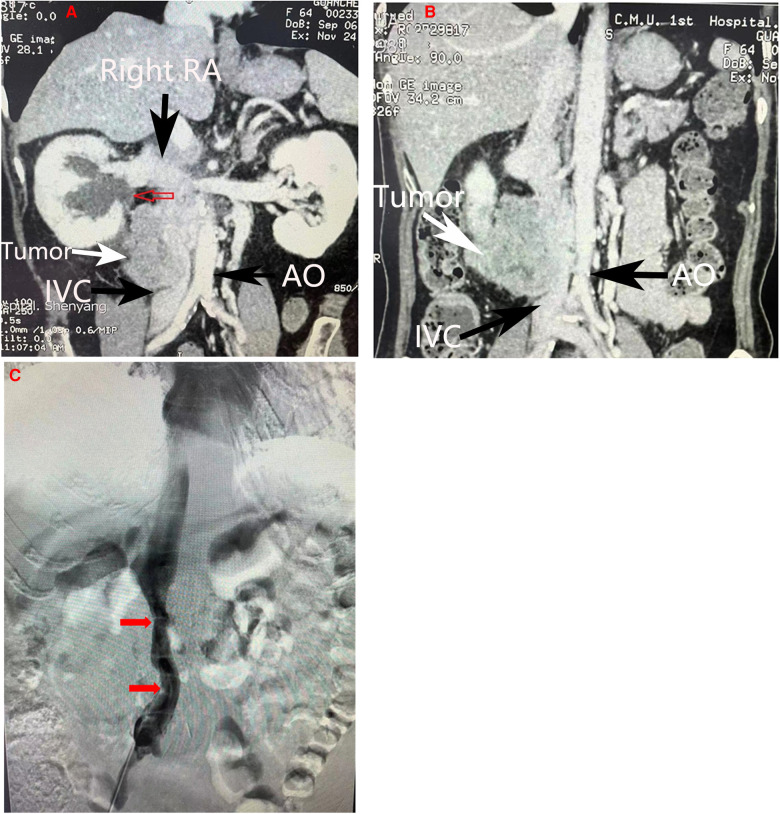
Imaging manifestations. (A) Coronal section of abdominal CT scan demonstrating compression of the right ureter with proximal ureteral dilatation and hydrops(red arrow). (B) Coronal section of abdominal CT scans howing a heterogeneous mass invading the inferior vena cava. (C) Inferior vena cava angiography revealing compression and narrowing of the inferior vena cava(red arrow). The main vessels were marked by arrows in other colors. RA, Right artery; IVC, inferior vena cava; AO, Aorta abdominalis.
